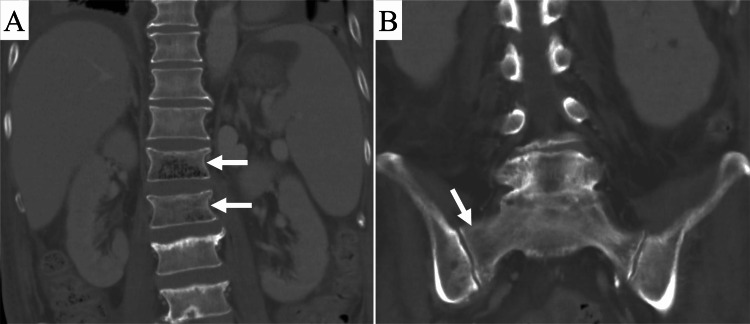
Computed tomography (CT) images of the lumbosacral spine obtained 118 days after liver transplantation. (A) Coronal CT image of the lumbar spine shows a decrease in intraosseous gas (arrows) in the first and second lumbar vertebral bodies. No extraosseous gas is observed. (B) Coronal CT image of the sacrum shows no intraosseous gas in the right sacral ala (arrow).
